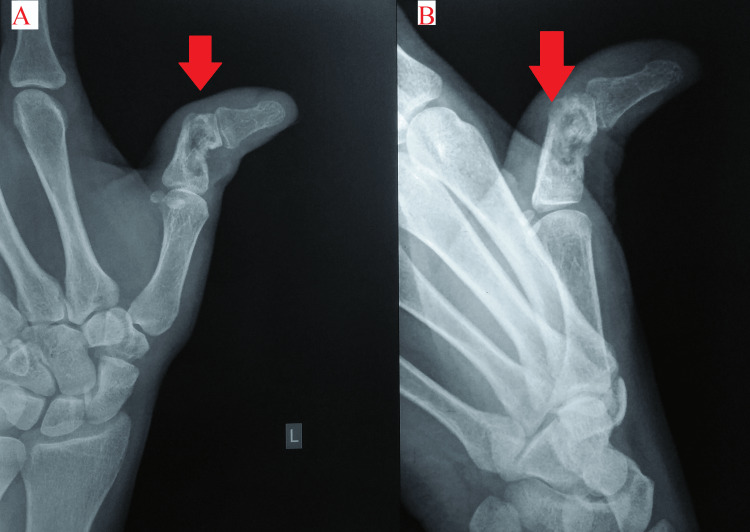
Plain radiographs of the left hand showing swollen left thumb with lesions. A: Anteroposterior view. B: Oblique view.
